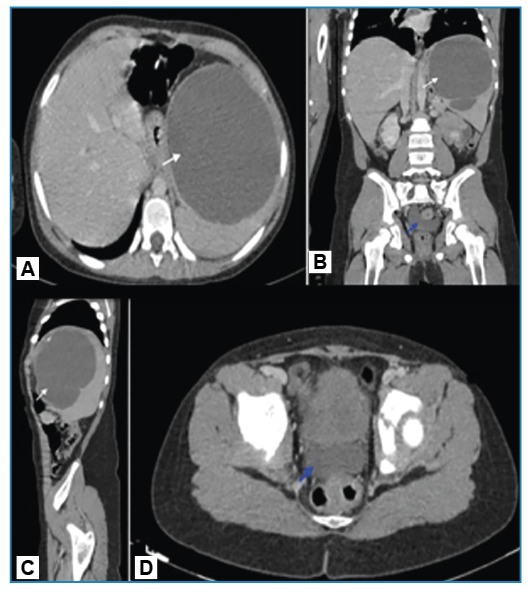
Axial- (A), coronal- (B) and sagittal-plane (C) abdominal CT showing a thick-walled hypodense cystic lesion approximately 11 cm in diameter in the spleen, with septations and wall calcification in the superior aspect (white arrows); axial- (D) and coronal-plane (B) abdominal CT showing free fluid of approximately 2 cm depth in the pelvic area (blue arrow).
